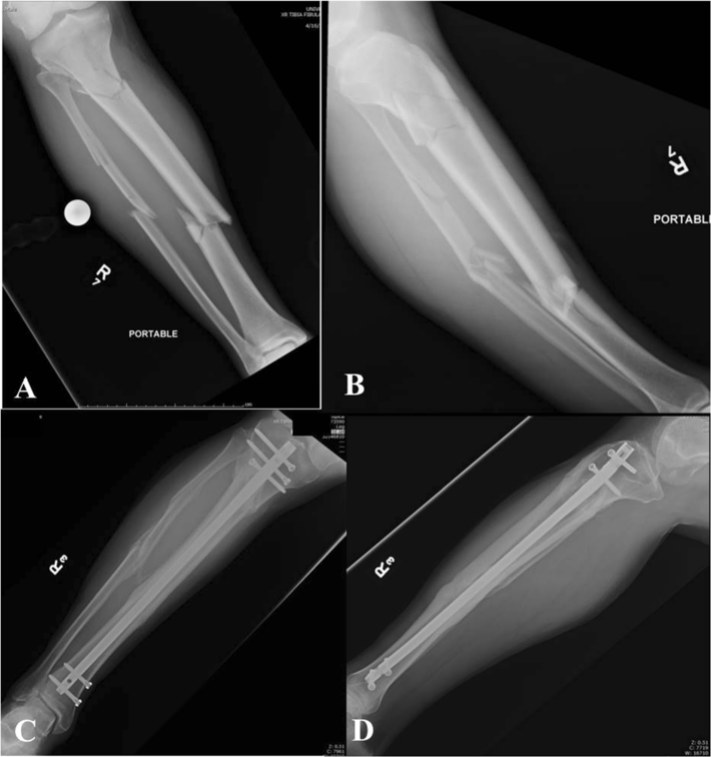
a-d Segmental tibia fracture (a-b) treated with intramedullary nailing with two distal and three proximal interlocking screws. Follow-up radiographs (c-d) demonstrate uneventful healing
